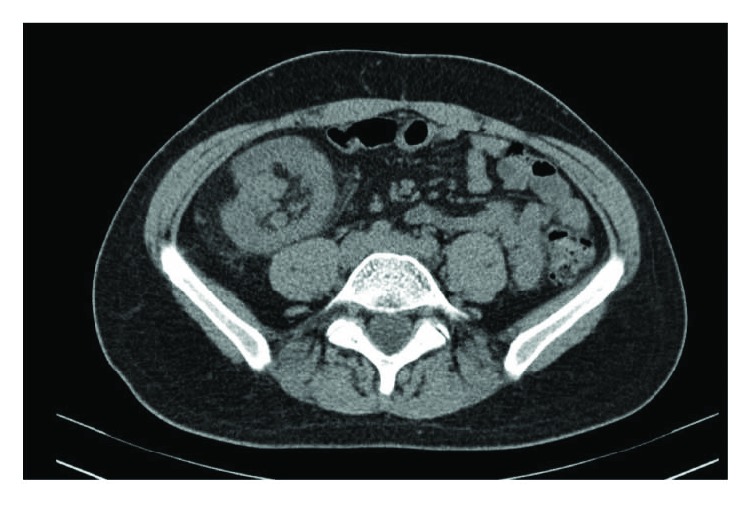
CT showed a hyperdense 60 × 50 mm right colic parietal lesion, signs of ileocolic intussusception with adjacent lymphadenopathy.
